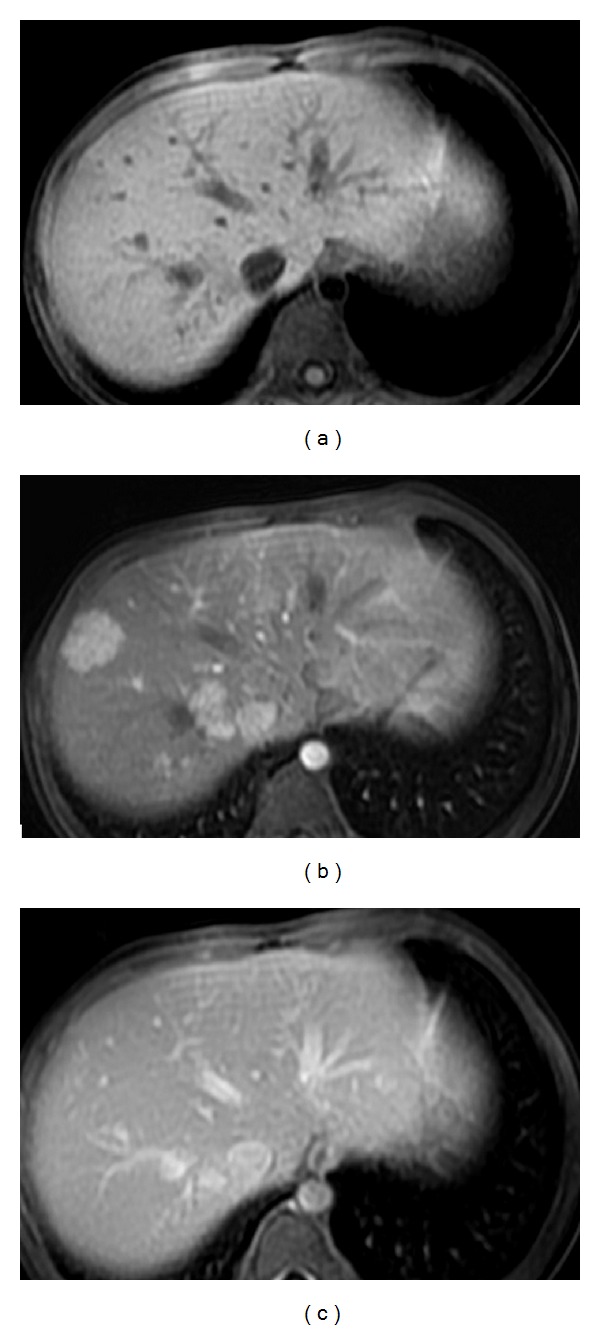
Nine-year-old girl with a history of metastatic right nephroblastoma treated with chemo- and radiotherapy. Liver MRI with T1-weighted images without (a) and with contrast injection at the arterial (b) and portal phases (c), performed six years after the end of treatment, displays multiple hepatic nodules, from 1 to 3 cm large, that enhance strongly after contrast injection at the arterial phase (b) and have almost the same signal as the surrounding liver before contrast injection (a) and at the portal phase (c). Simple clinical and imaging followup was performed (courtesy of Dr. H. Brisse, Institut Curie Paris, France).
